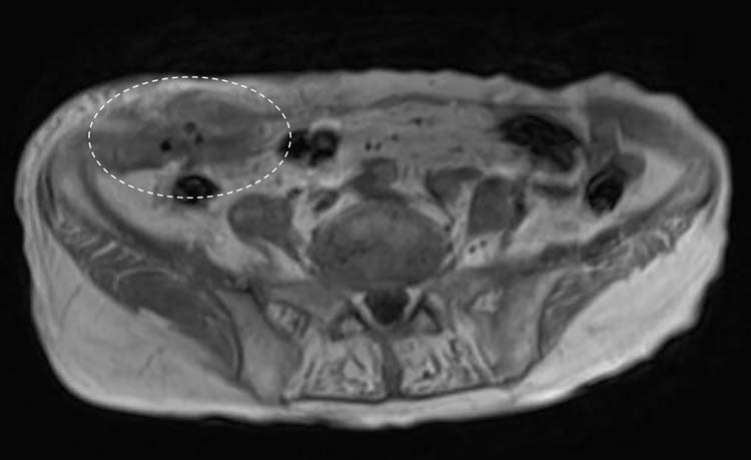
Abdominal MRI showed a low-signal nodule on T1-weighted image (encircled).
